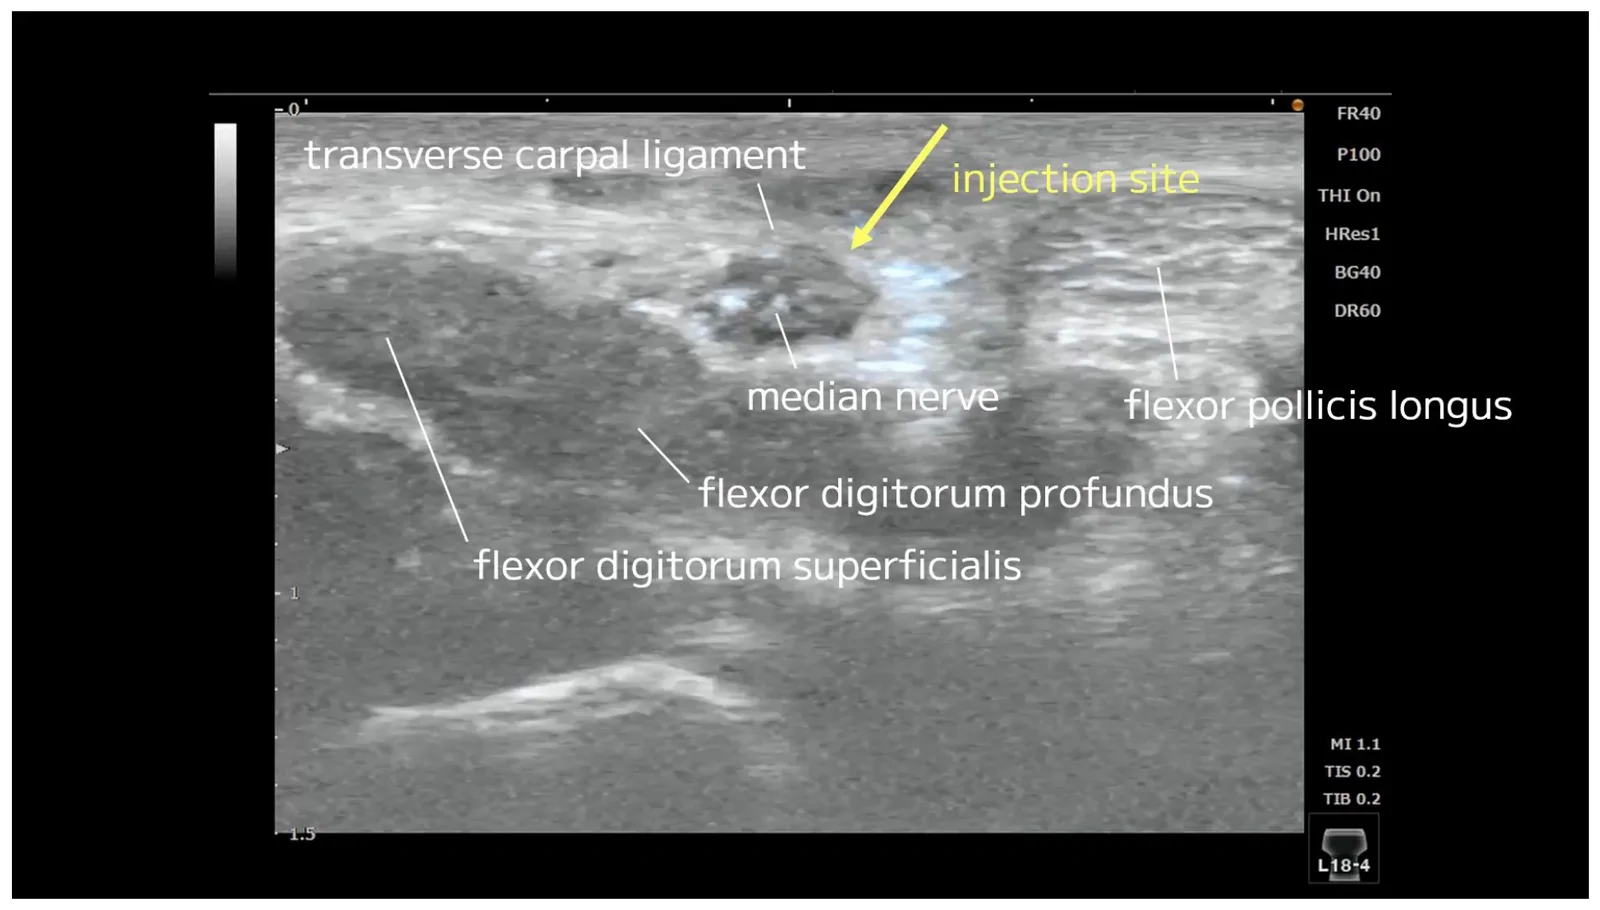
Point 11—median nerve/transverse carpal ligament: ultrasound-guided fascia hydrorelease; yellow arrow indicates the injection site.
